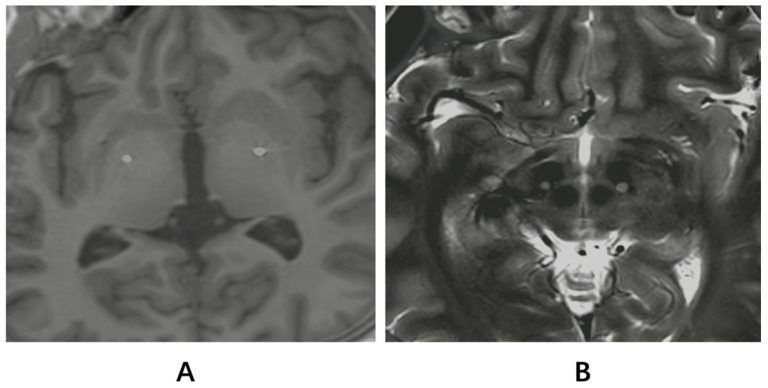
Postoperative electrodeposition. (A) The figure shows the fusion of the postoperative CT and preoperative T1-weighted MR images of one person using the Matlab SPM tool. (B) The figure shows the fusion of the postoperative CT and preoperative T2-weighted MR images of one person using the Matlab SPM tool. (A): GPi. (B): STN.
